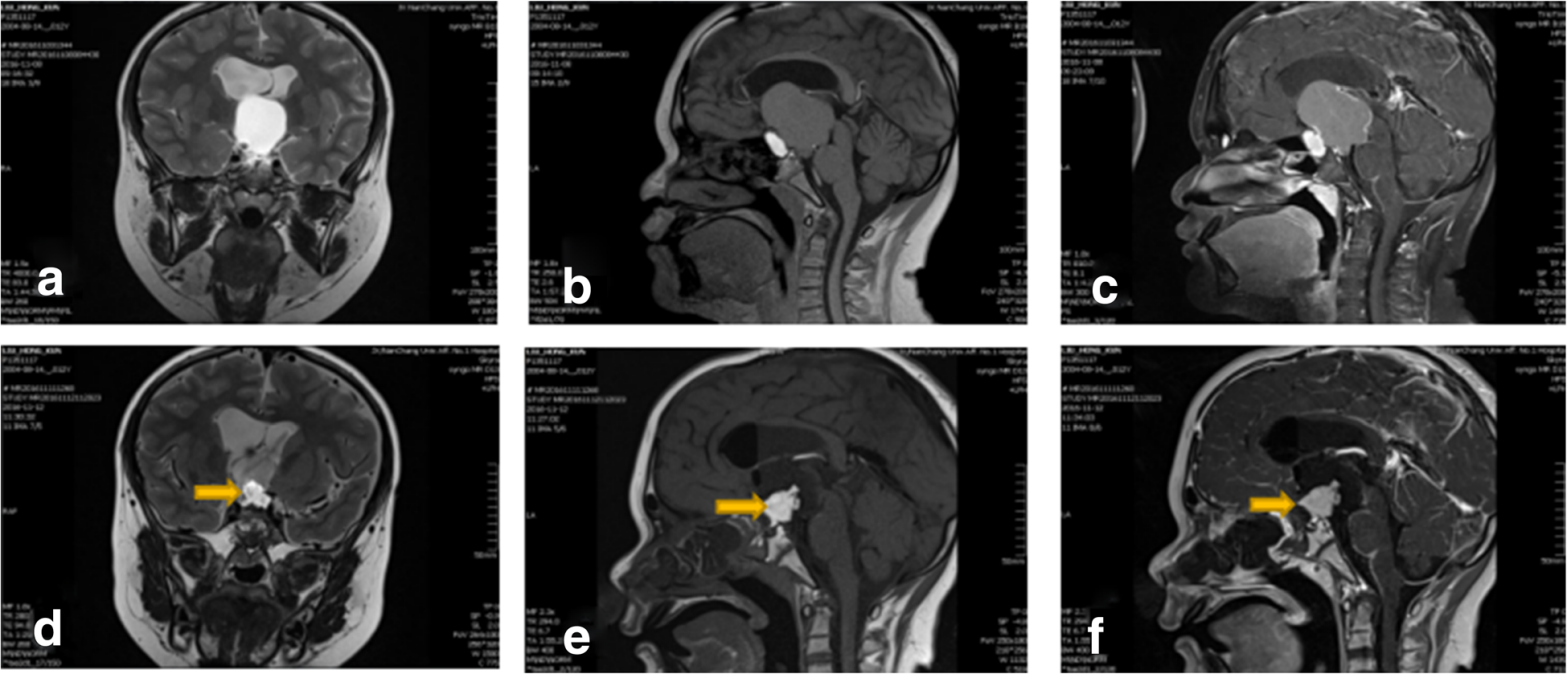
The pre- and post - operative MR images. a, b, c: coronal T2-weighted magnetic resonance image (a) and sagittal T1-weighted MR image (b) and sagittal T1-weighted MR with contrast image (c) taken before operation showed a huge cyst-solid craniopharyngioma lesion; d, e, f: coronal T2-weighted MR image (d) and sagittal T1-weighted MR image (e) and sagittal T1-weighted MR with contrast image (f) taken on postoperative day 1 showed that the tumor were total resection. The Yellow arrows showed auto-adipose tissue for repairing diaphragma sellae after tumor section
